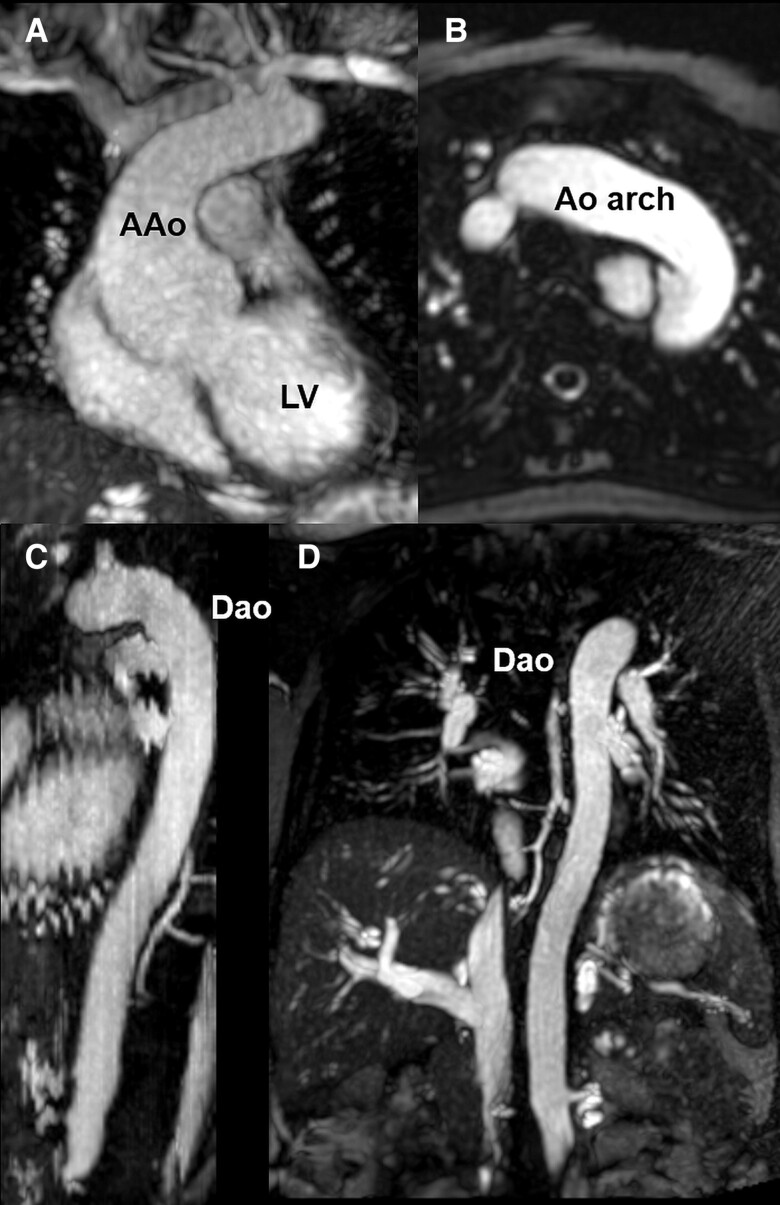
Cardiovascular magnetic resonance images without contrast at 21 weeks of gestation showing the ascending aorta, aortic arch, and descending aorta (*A–D*). AAo, ascending aorta; Dao, descending aorta; LV, left ventricle.
